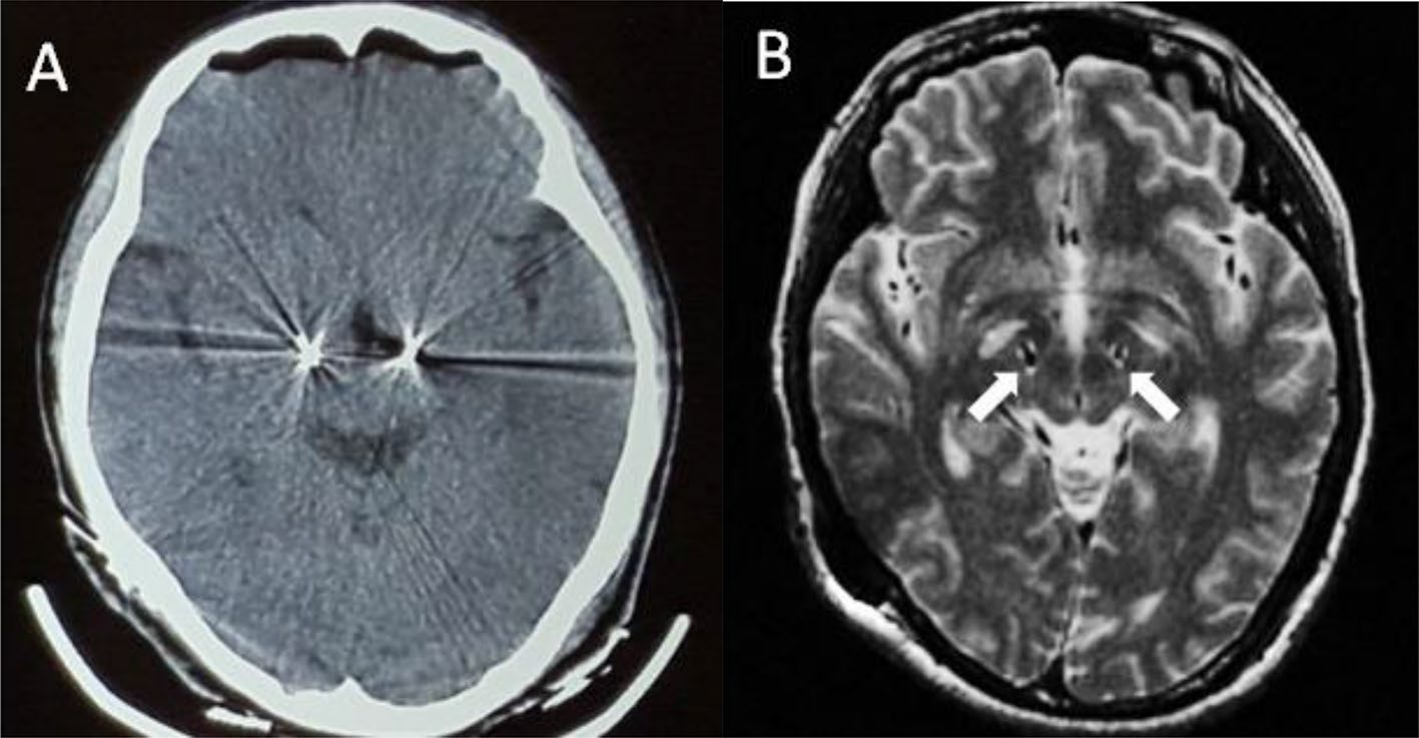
Case 4. Postoperative brain CT (A) for ruling out haemorrhage and axial T2-weighted MR images (B) for showing the targets location (white arrow) of the subthalamic nucleus.
